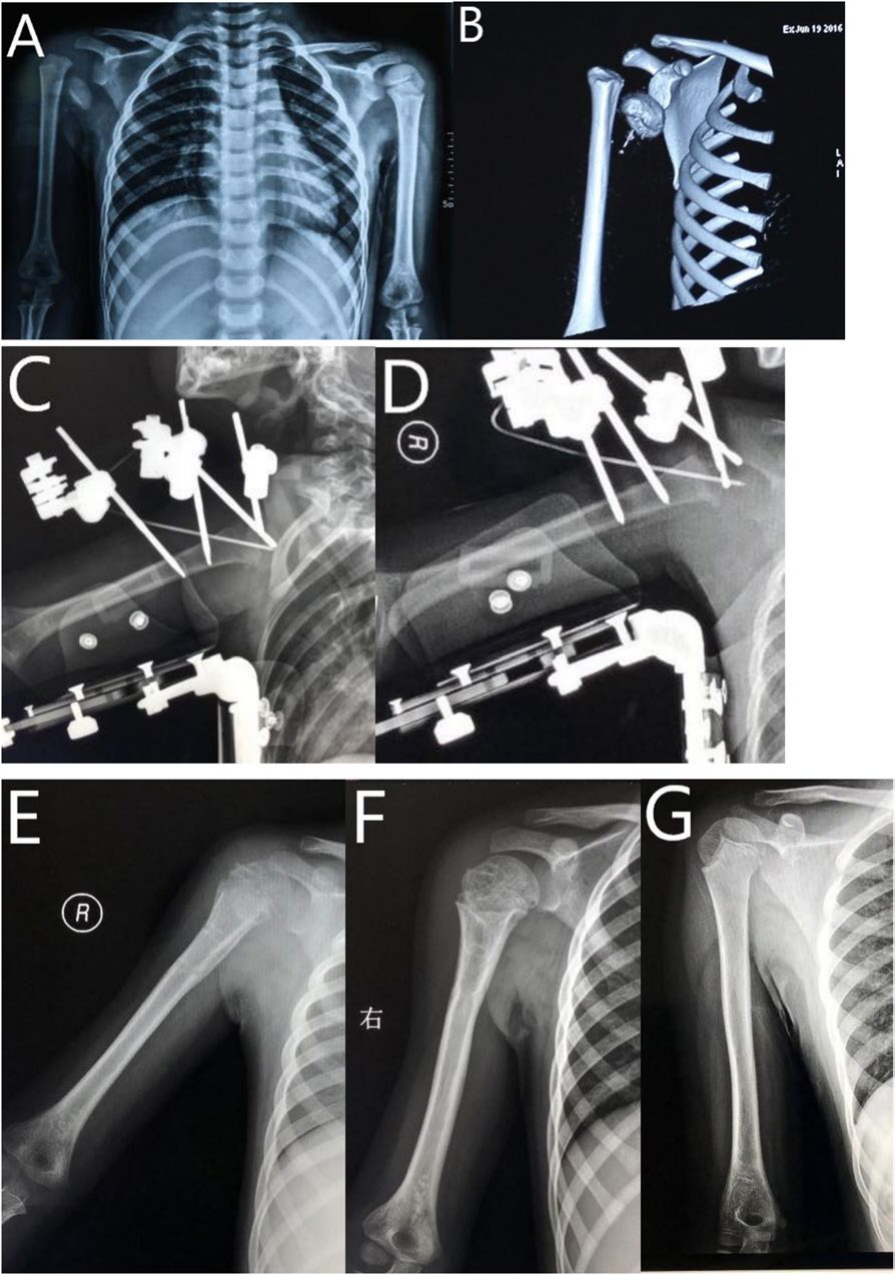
5-year-old boy with proximal humeral epiphyseal fracture SH I and shoulder dislocation. A Radiograph of humerus before surgery. B 3D reconstruction of CT scan. C Radiograph of the humerus after surgery. D Radiograph of the humerus at 1-month follow-up. E Radiograph of the humerus at 3-month follow-up. F Radiograph of the humerus at 6-month follow-up. G Radiograph of the humerus at 4-year follow-up
